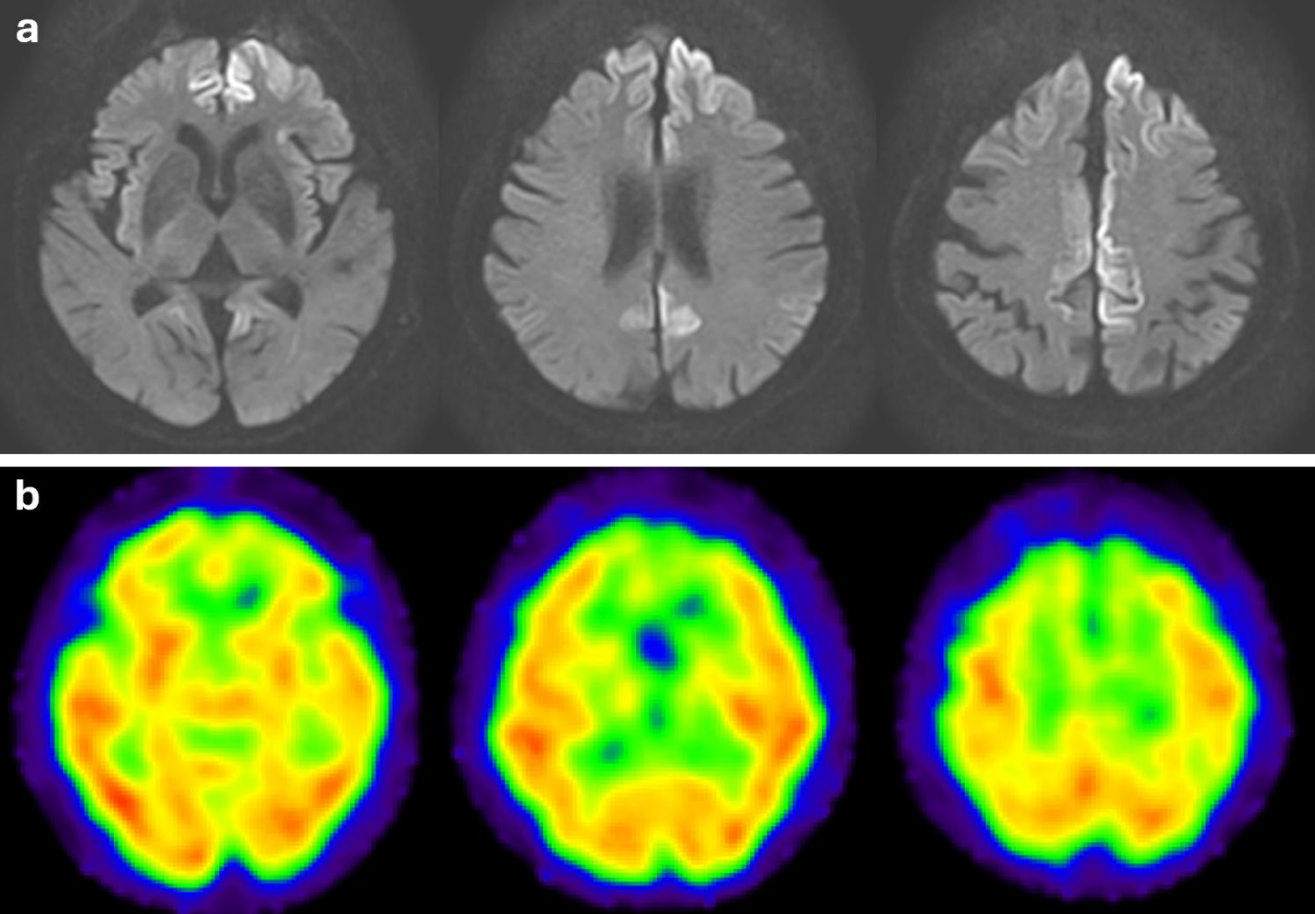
An 87-year-old male with progressive cognitive impairment diagnosed with Creutzfeldt–Jakob disease. a Axial DWI showed a high-intensity area along the cortex, and b IMP brain perfusion images revealed hypoperfusion in the same area
